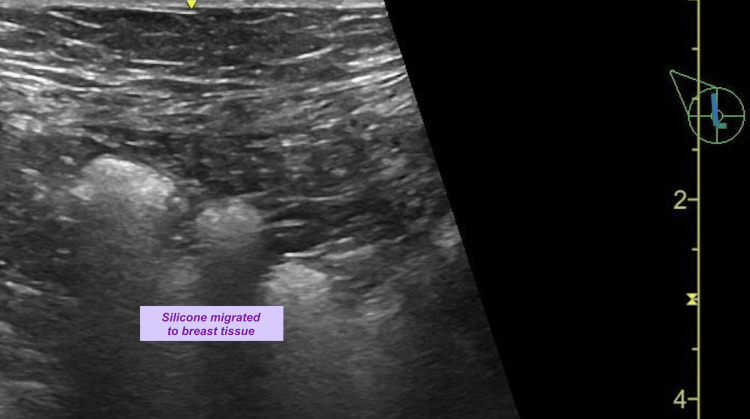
Breast ultrasound demonstrating the characteristic "snowstorm" appearance adjacent to the right implant, indicative of extracapsular silicone leakage
